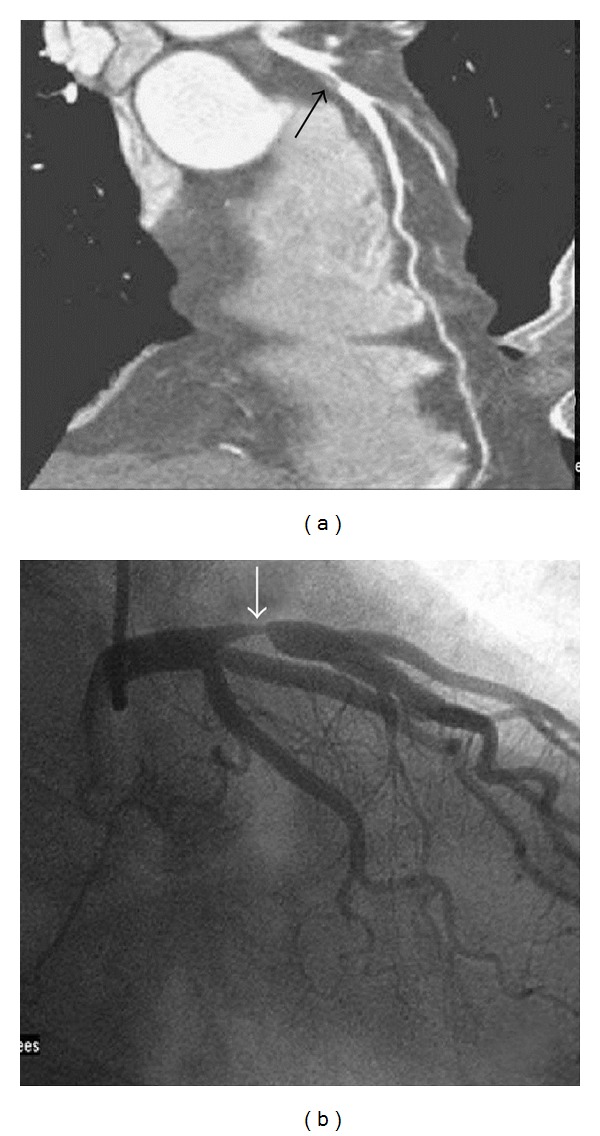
A 53-year-old male with zero calcium score. (a) A computed tomographic angiogram shows a soft plaque at the left anterior descending artery (LAD) (black arrow) with severe stenosis. (b) Conventional coronary arteriogram confirms the severe stenosis over the proximal third of the LAD (white arrow).
